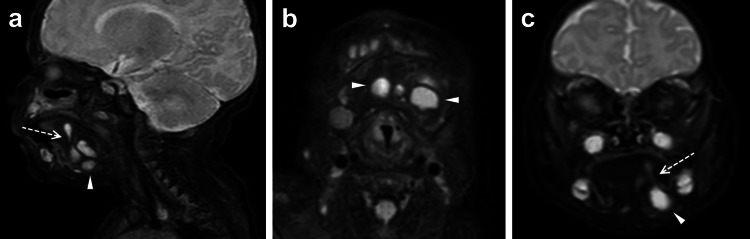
(a) Sagittal, (b) axial, and (c) coronal T2-weighted postcontrast MRI images demonstrated a multiseptated cystic lesion (arrowheads) in the submandibular space. A tail (dashed arrows) extends from the sublingual space and communicates with submandibular space, consistent with a plunging ranula.
